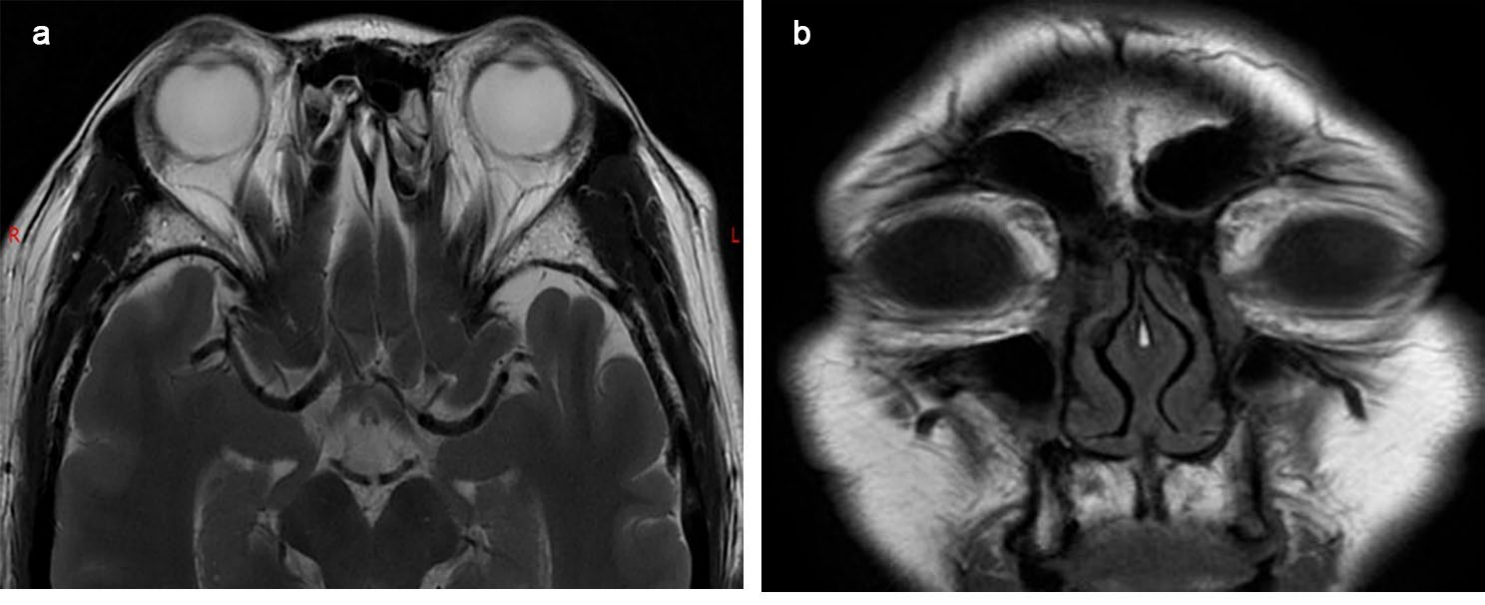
MRI images of the orbit. (A) Transverse orbital T2-weighted image (T2WI) showing slight thickening of the soft tissue of both eyelids. (B) Enhanced orbital scan showing slight thickening of the soft tissues of both eyelids and no obvious abnormality in the bilateral lacrimal glands
